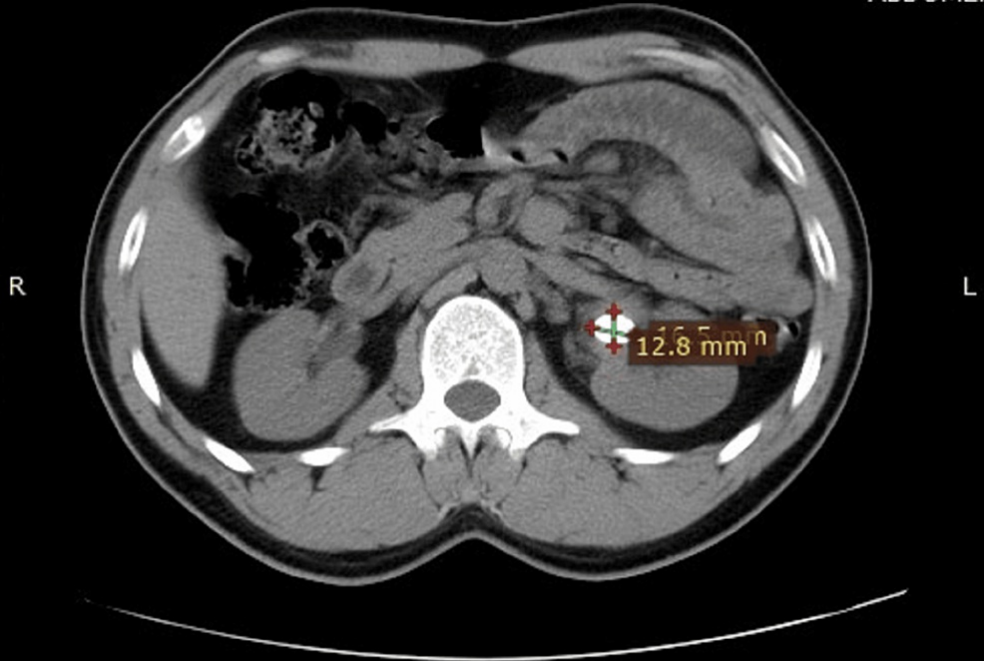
Preoperative CT KUB image showing a calculus in the left kidney (coronal view). CT KUB = computed tomography of the kidneys, ureters, and bladder
